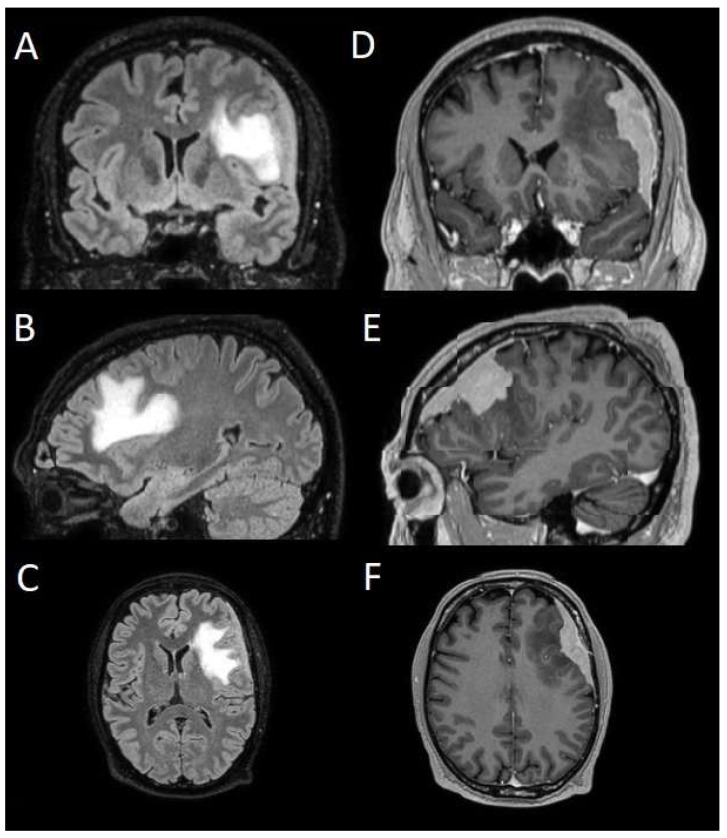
Sequences selected for the segmentation. (A–C) Coronal, sagittal, and axial FLAIR sequences to quantify PE. (D–F) Coronal, sagittal, and axial T1-weighted sequences with contrast enhancement to estimate meningioma volume. FLAIR: fluid-attenuated inversion recovery; PE: peritumoral edema.
